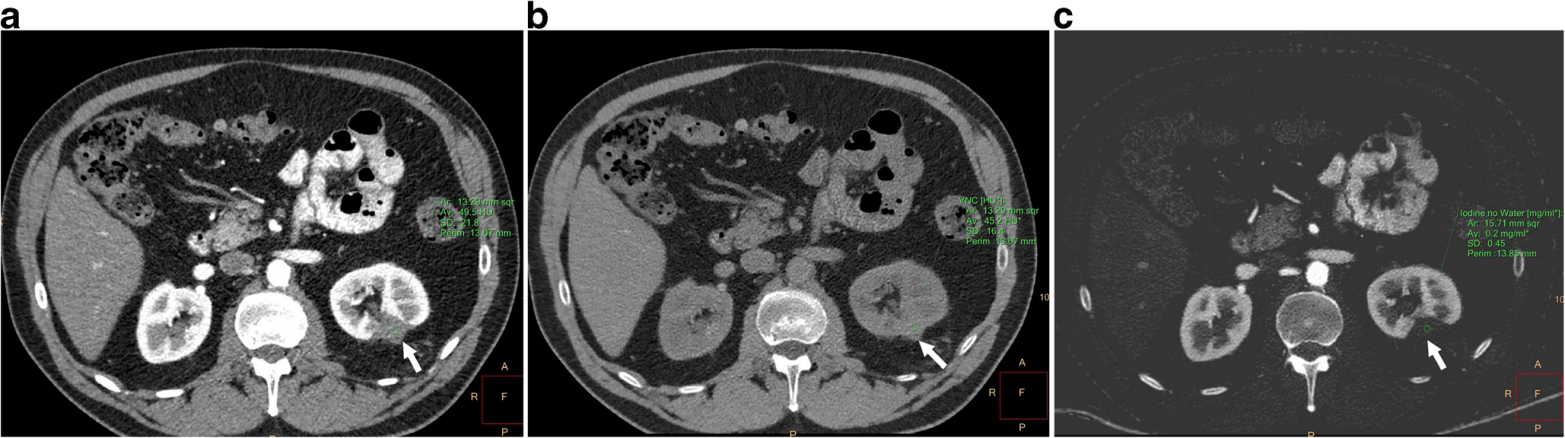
Lesion characterisation. (a) 120-kVp routine-diagnostic (conventional) CT image through the upper abdomen shows a well-defined hypoattenuating lesion in the left kidney (*arrow*), which has a mean attenuation of 49.5 HU, which is too high for a simple cyst. This can either be a complicated cyst or enhancing solid lesion. (b) VNC reconstruction at the same level shows that the attenuation of the lesion (*arrow*) is 45.2 HU, indicating that the high attenuation was inherent in the scan and not due to contrast administration (c) Iodine map at the same level confirms the above, with an insignificant (0.2 mg/ml) iodine uptake in the lesion (*arrow*). This constellation of findings helps in diagnosing this as a complicated renal cyst than an enhancing solid mass
